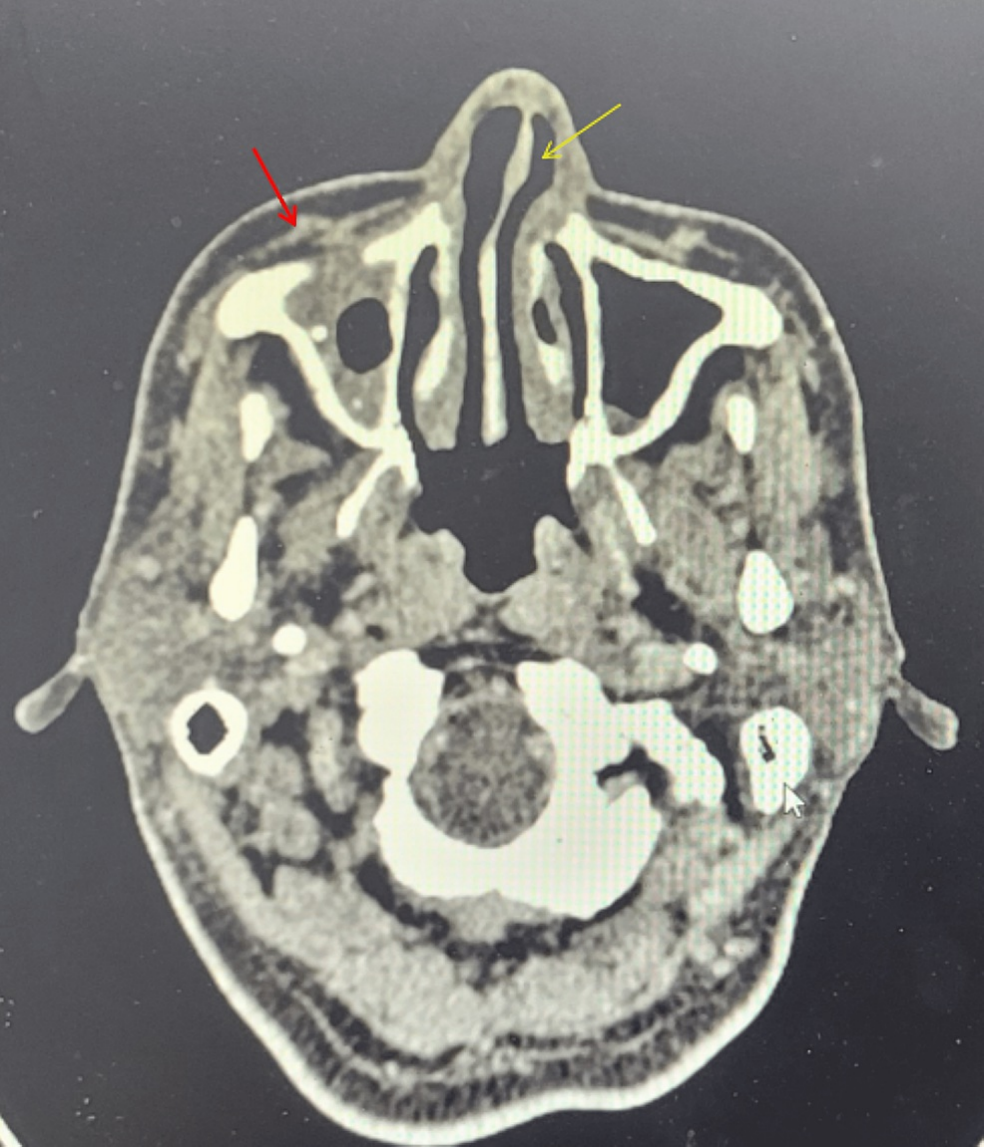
Computed tomography axial section showed median nasal septal deviation with opacification within the right maxillary sinus and destruction of the anterolateral wall of the right maxillary sinus.
